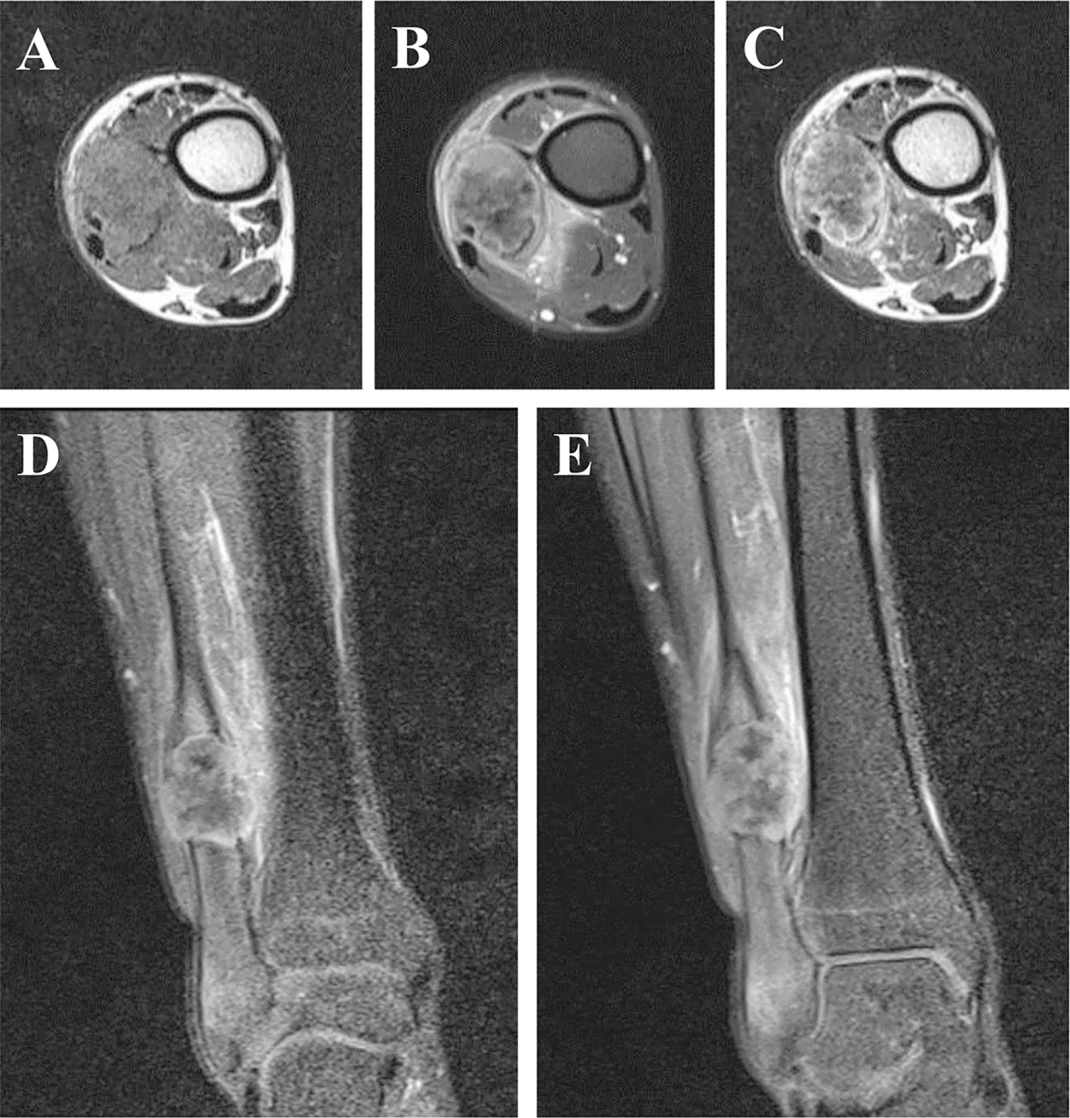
Axial T1-weighted image without contrast enhancement. (A), T2-weighted image (B) and the arterial phase of T1-weighted image (C). The arterial (D) and the venous (E) phase of coronal T1-weighted images. MRI revealed a focal, hypo- and isointense mixed mass on T1-weighted image (A), and hypo- and hyperintense mixed mass on T2-weighted image (B). There were no signs of necrosis, hemorrhage, or cyst formation within the mass. However, adjacent soft tissue edema and swelling was seen. Gadolinium-enhanced T1-weighted images revealed obvious perilesional enhancement, particularly in the region adjacent to the normal tissue (C–E).
